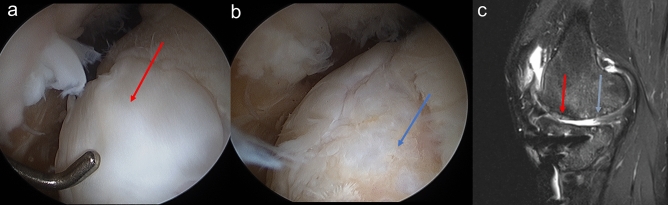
Second-look arthroscopic findings of (a) the anterior lesion of MFC (ICRS Grade I) and (b) the mid to posterior lesion of MFC. (ICRS Grade IIIb) (c) At the 1-year follow-up, the anterior repaired cartilage (red arrow) completely filled the defect, but mid to posterior cartilage (blue arrow) showed an irregular surface and poor integration with incompletely filled defect at T2 PD FS MRI images.
